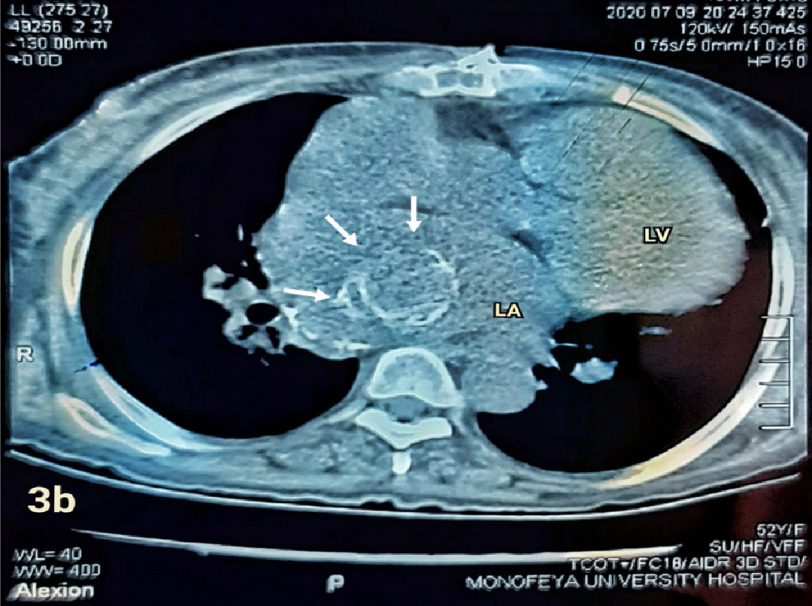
Computed tomography of the patient showed dilated cardiac chambers and round mass in the LA.
